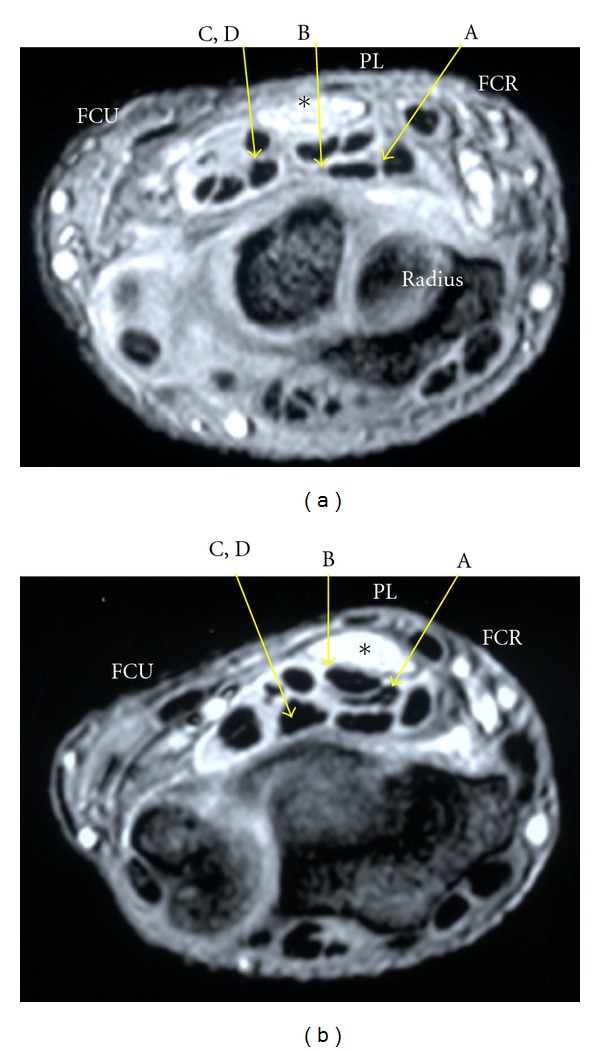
(a) Wrist of a patient with severe CTS at the level of the distal part of the distal radioulnar joint. The median nerve is enlarged and can be seen as a high-intensity area on the MRI. Insertion from the entry point close to the ulnar side of the PL results in contact between the needle and the nerve. Insertion from C and D leaves a narrow margin between the needle and the nerve. *Median nerve. (b) Wrist of a patient with extremely severe CTS. At the level of the distal part of the distal radioulnar joint, the median nerve is enlarged and can be seen as a high-intensity area on the MRI. Insertion from the entry point close to the ulnar side of the PL and FCR results in contact between the needle and the nerve. *Median nerve.
